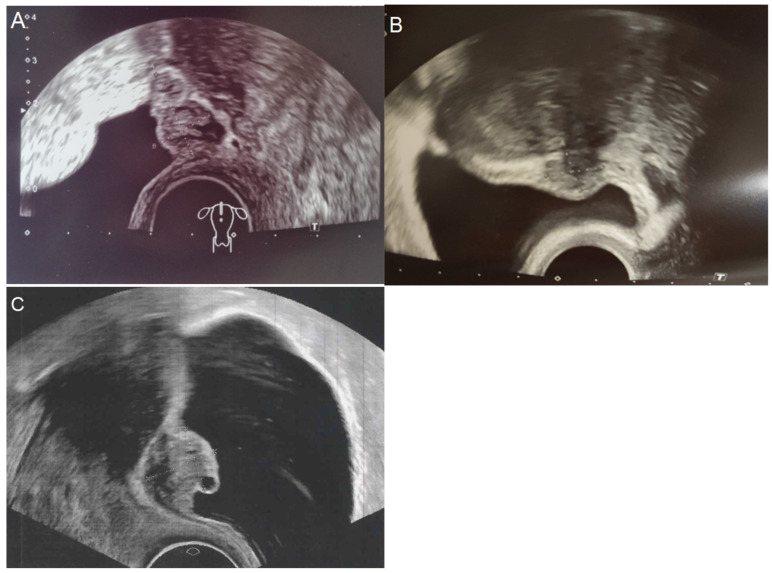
Transvaginal sonography. (A) The endometriosis nodule is located on the bladder dome and the bladder base. (B) The endometriosis nodule is located on the bladder dome, far from the bladder base. (C) The endometriosis nodule is located on the bladder dome, close to the bladder base.
